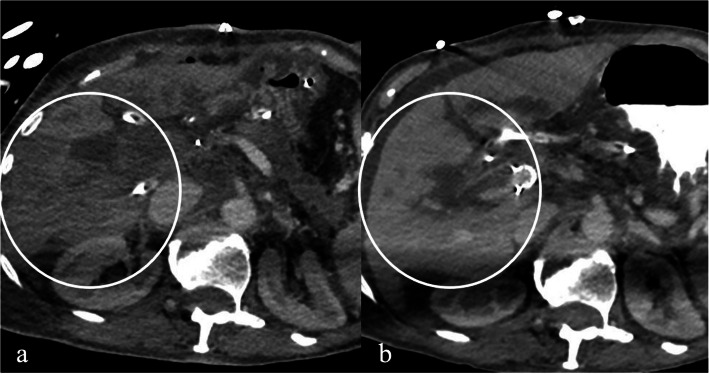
a) CT scan prior to portal vein stent confirming portal vein occlusion and transient hepatic attenuation differences in the early portal phase (circle). b) Liver perfusion significantly improved with no further attenuation differences (circle) and patent portal vein
